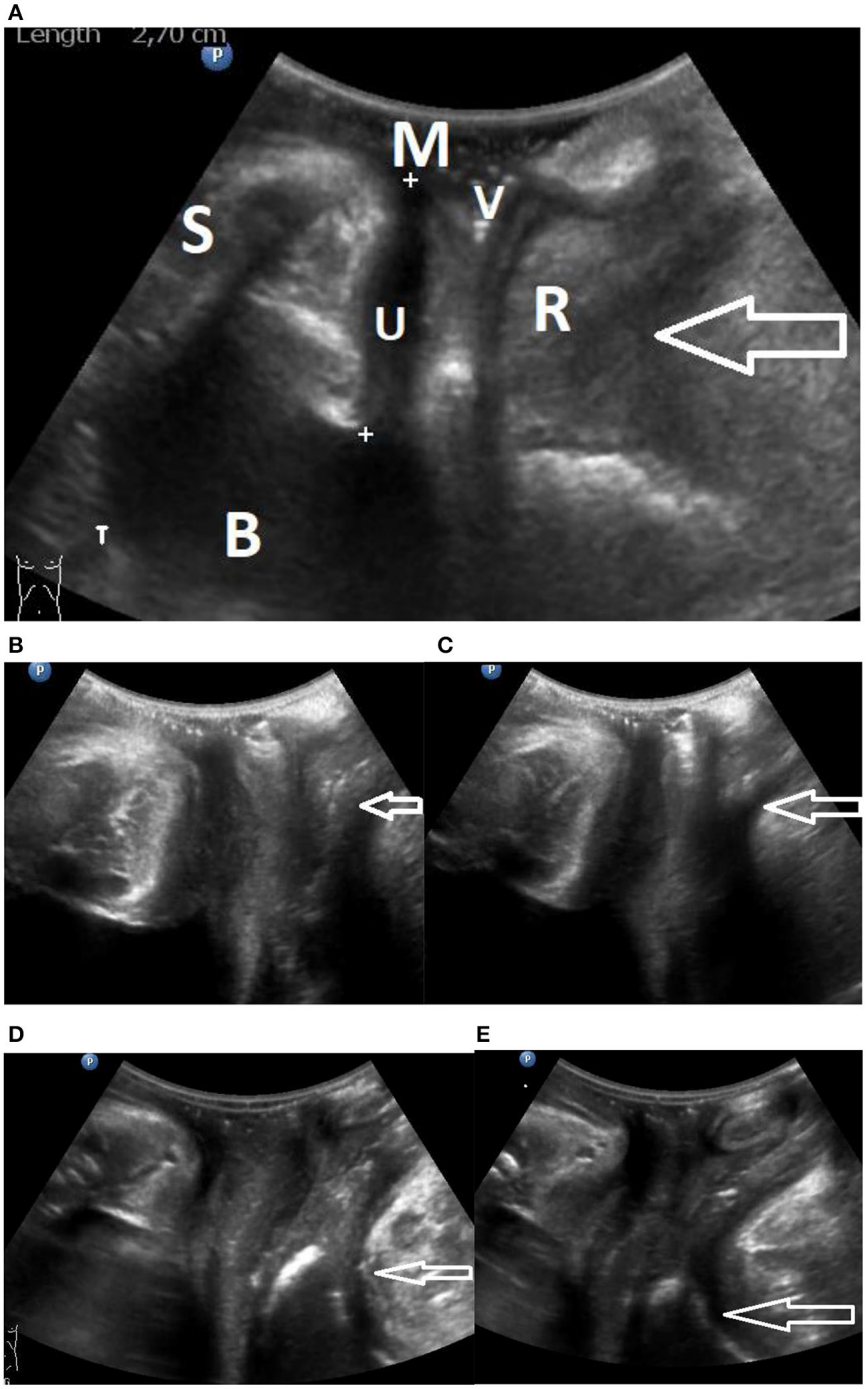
(A) Gives an overview of a perineal ultrasound image. B, bladder; U, urethra; V, vagina; R, rectum; M, meatus; S, symphysis. The point of the arrow is in the puborectalis muscle. (B,C) Snapshots from a video relaxed and contracted. Distal engagement of the PRM, (B) relaxed and (C) contracted state, arrows point at PRM engagement. Videos are uploaded as proximal video and distal video. (D,E) Snapshots from a video relaxed and contracted. Proximal engagement of the PRM, (D) relaxed state, (E) contracted state. Arrows point at PRM engagement.
